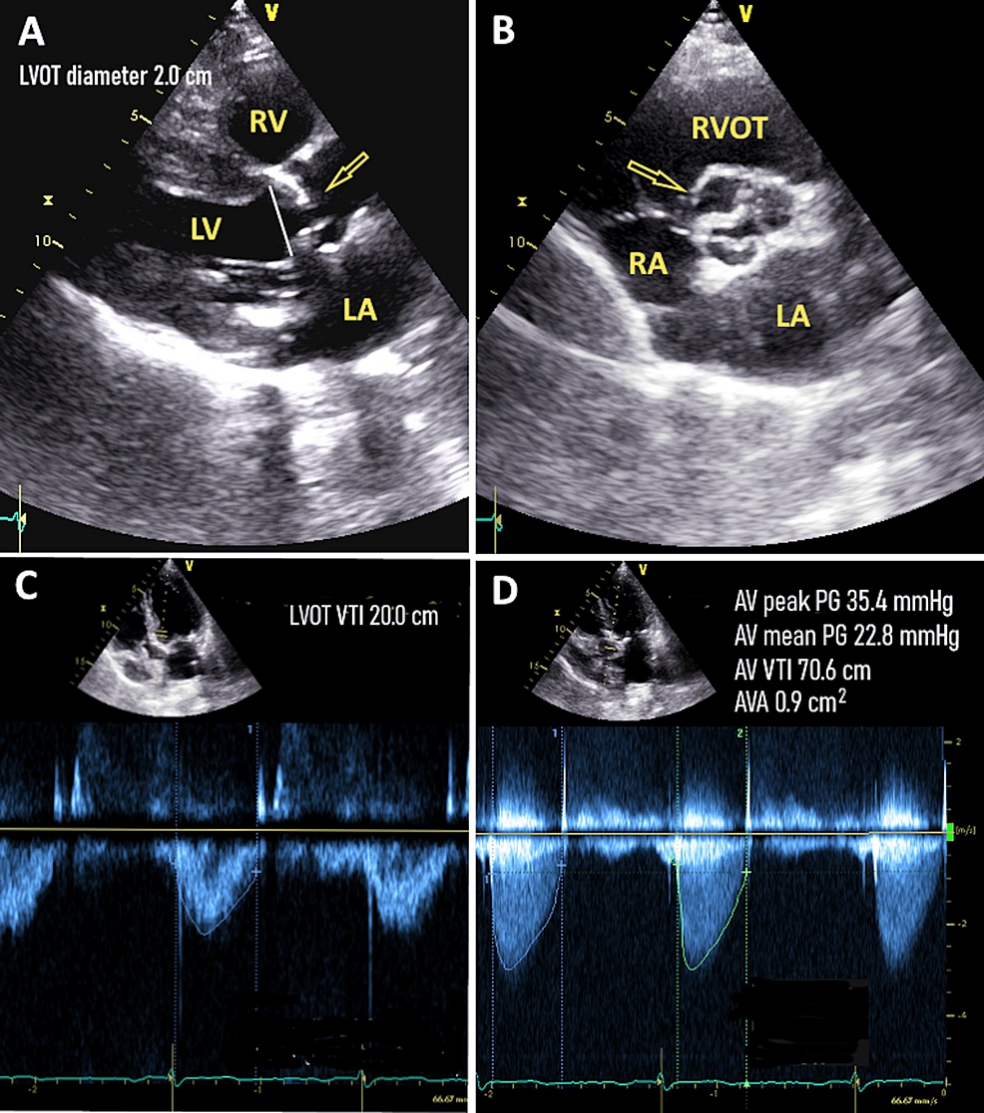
An example of the echocardiographic assessment of a 90-year-old lady with preserved left ventricular function and low-gradient severe aortic stenosis (A) Parasternal long axis view. A calcified aortic valve with a limited opening is seen (arrow). LVOT diameter was measured at 2.0 cm. (B) Parasternal short axis view. A calcified aortic valve with a limited opening is shown (arrow). (C) A pulsed wave Doppler through the left ventricle outflow tract at the five-chamber apical view is seen. (D) Continuous wave Doppler through the aortic valve at the five-chamber apical view is shown. The echocardiography examination of this patient is consistent with low-gradient severe aortic stenosis. Her weight was 71 kg, her height was 162 cm, and her BSA was calculated at 1.79 m². The stroke volume index was 35.3 ml/m² (borderline). The flow rate through the aortic valve was 196.8 ml/s (low). LV: Left ventricle; RV: Right ventricle; LA: Left atrium; RA: Right atrium; LVOT: Left ventricle outflow tract; RVOT: Right ventricle outflow tract; VTI: Velocity time integral; AVA: Aortic valve area; AV peak PG: Aortic valve peak pressure gradient; AV mean PG: Aortic valve mean pressure gradient.
